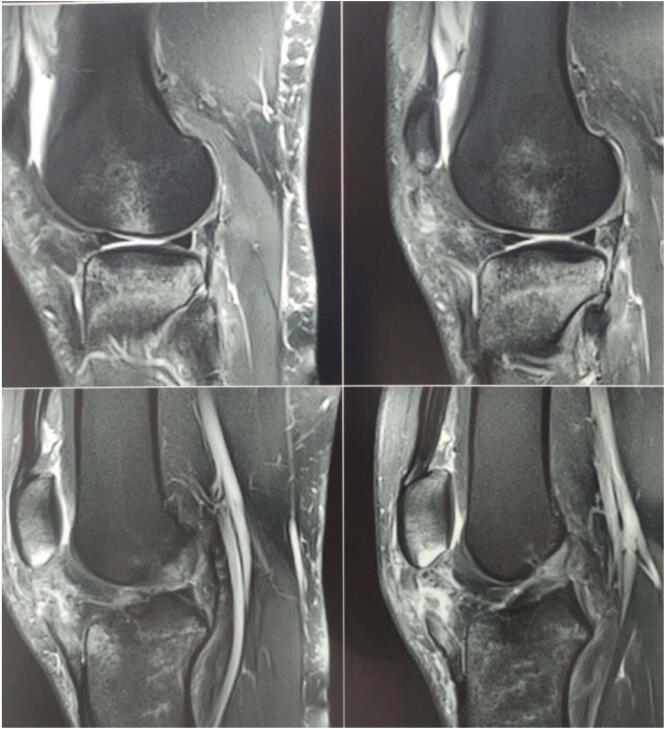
MRI: complete rupture of the patellar tendon and anterior cruciate ligament.
